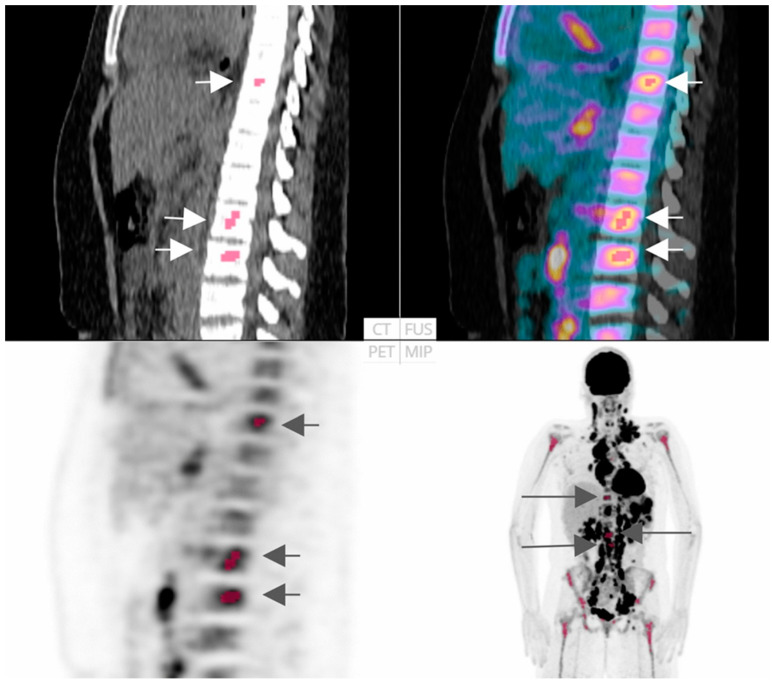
FDG-PET/CT representation: An instance where both AI-driven technique and the majority (8 out of 10) of physicians identified localized uptake in the skeletal/bone marrow (indicated in red and with arrows). Reprinted with permission from [30] under a Creative Commons Attribution 4.0 International License.
